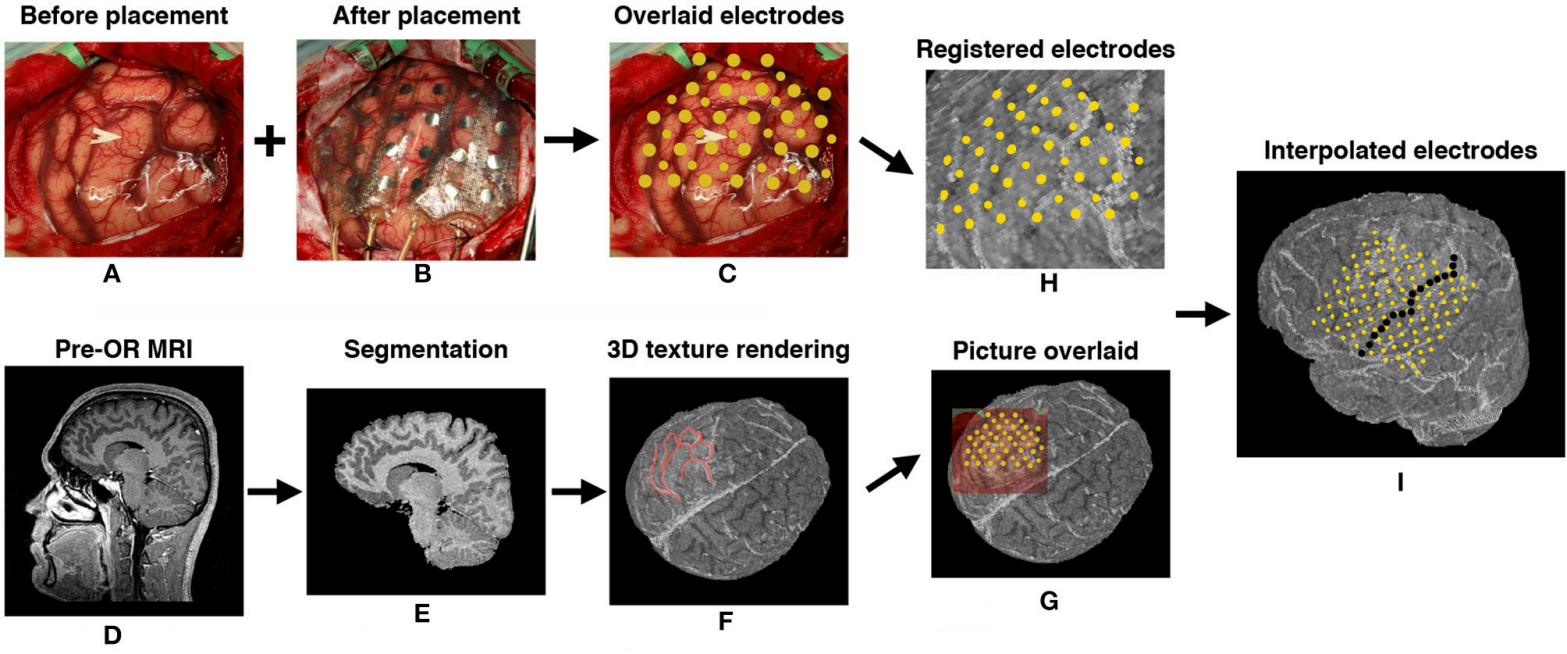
Intraoperative photo based electrode registration procedure for P2. (A) Cortex photo before electrode placement. (B) Photo taken from the same position after electrode placement. (C) Electrodes (disks in gold) manually segmented out and overlaid on the cortex. (D) Patient's preoperative MRI. (E) Gray and white matter segmentation. (F) 3D texture rendering of gray and white matter. Blood vessels were accentuated by red lines as landmarks. (G) Picture overlaid on 3D rendering by comparing the landmarks. (H) Electrodes registered on 3D rendering of the brain. (I) The side view of the cortex with registered and interpolated electrodes, central sulcus was delineated by a series of discrete black points.
